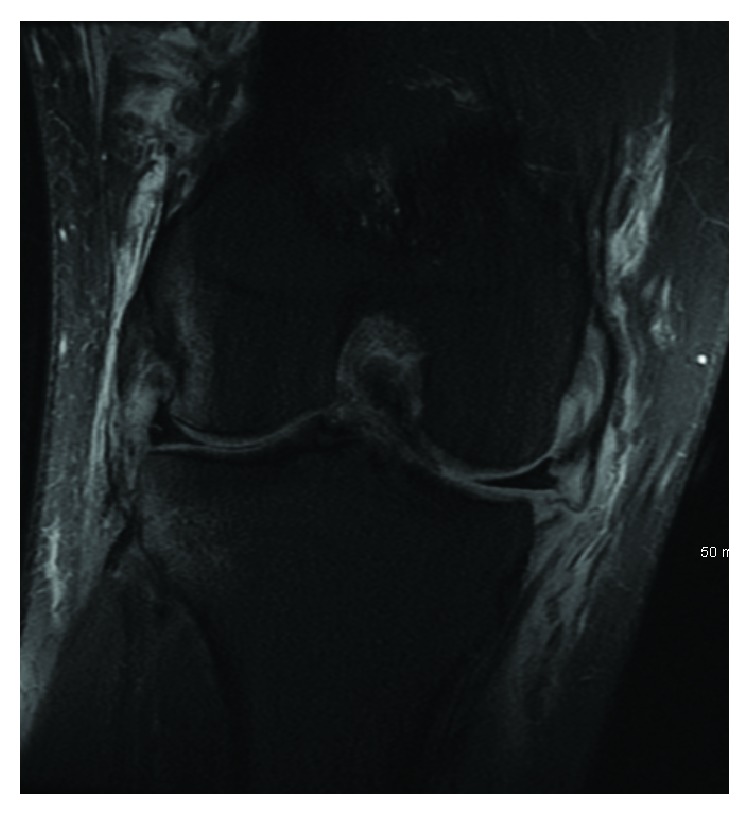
A T2 coronal MRI of the patient's right knee demonstrating a grade III MCL tear with partial extrusion of the medial meniscus.
